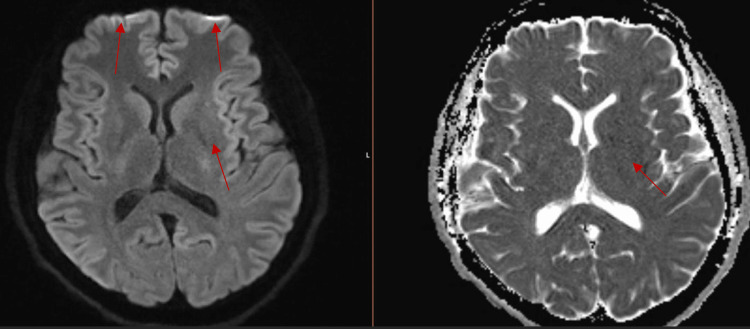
MRI T1/T2 Leptomeningeal enhancement of frontal lobes. Acute infarct in the left lentiform nucleus.
